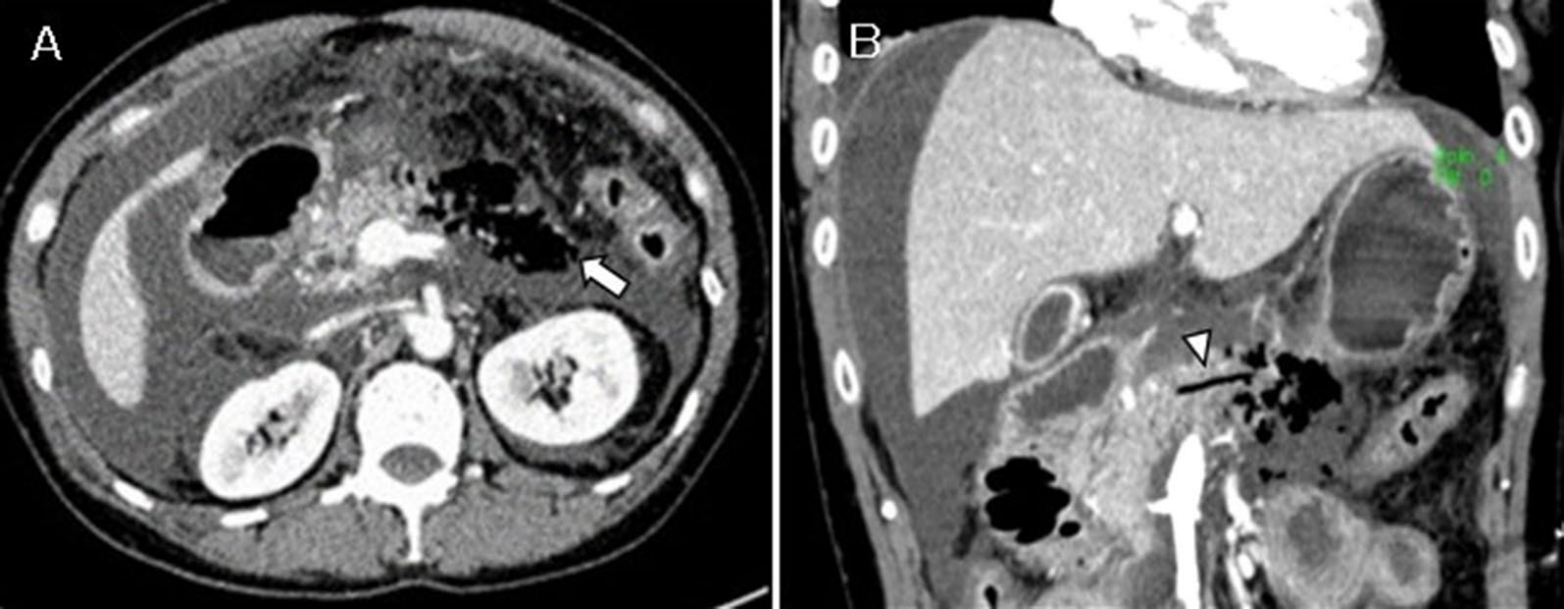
Contrast-enhanced CT of case 2. A It shows the emphysema around the pancreatic body including the retroperitoneal space (arrow). B Coronal view of the same CT scan showing emphysema in the pancreatic duct (arrowhead)
